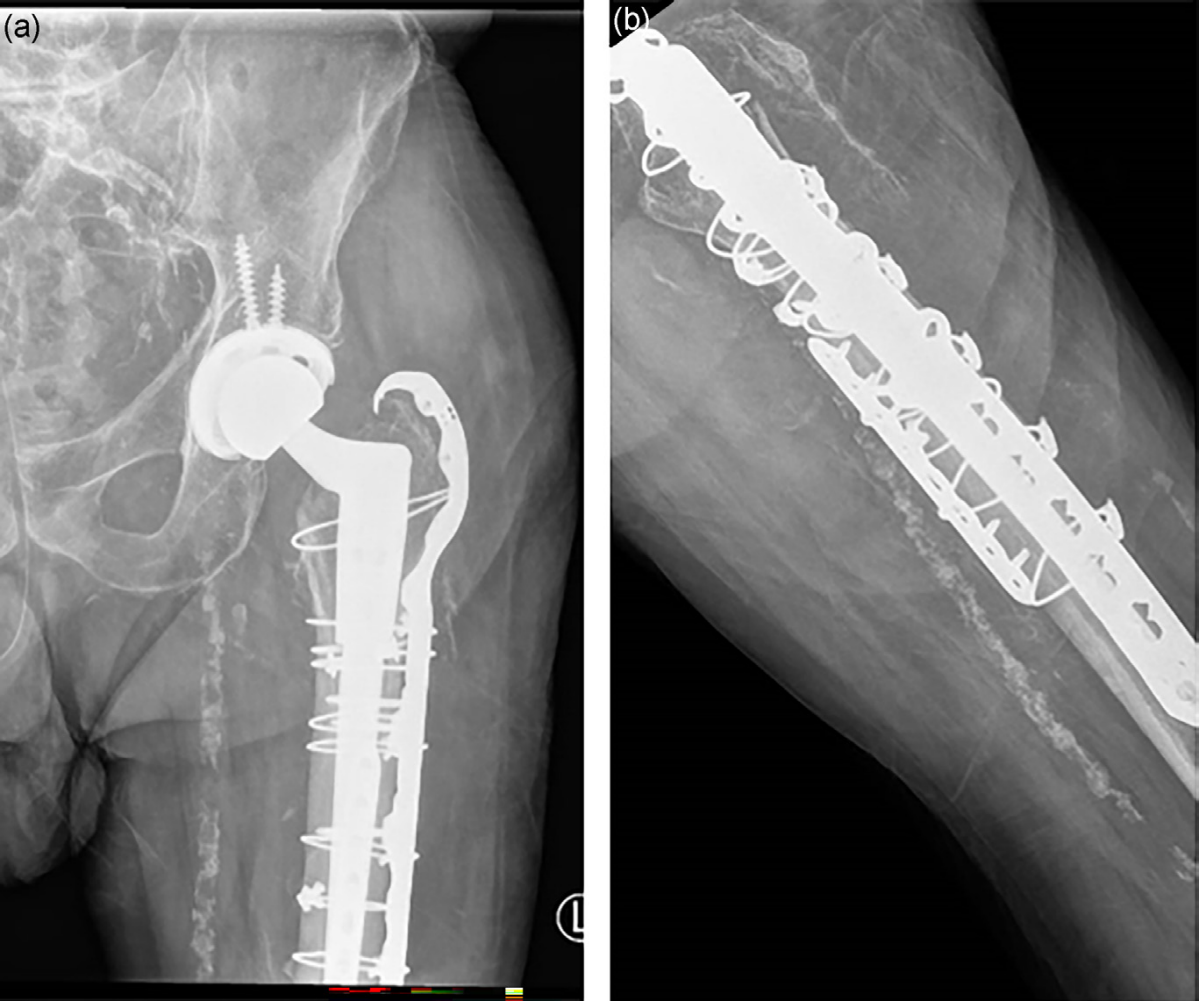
Post-operative L) hip x-ray following (a) revision and (b) fixation.
